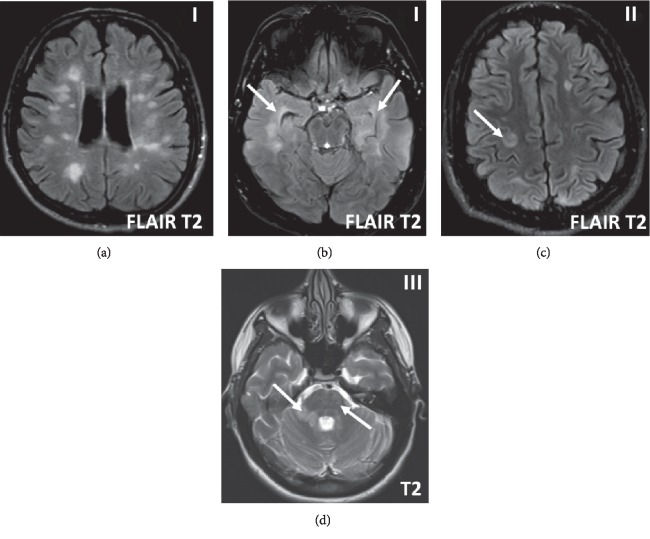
Onset disease axial FLAIR & T2 MR brain imaging. Heavy Periventricular zone (PVZ-I) lesion load (a & b). Note specific anterior temporal PVZ lesions (b, arrows). Cortical-juxta-cortical (zone II) lesion (c, arrow), other lesions are sub-cortical. Infratentorial (zone III) lesions in pons (d, subtle) and brachium pontis (large lesion right side (d, arrow).
